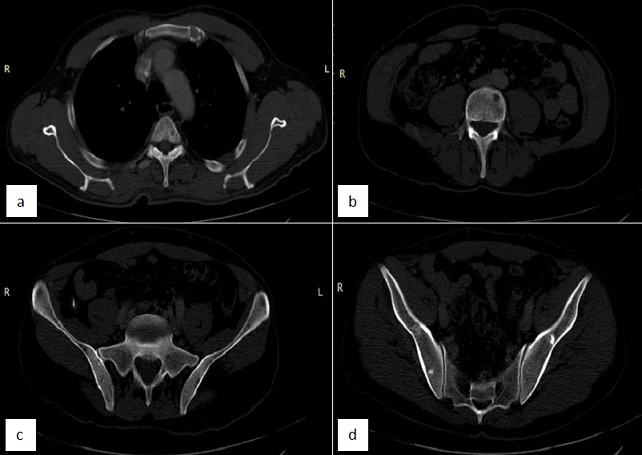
Cervical-thoracic-lumbo-sacral and pelvis CT scan: (A, B, C, D) diffuse lytic lesions and sclerotic bony lesions in iliac bones
